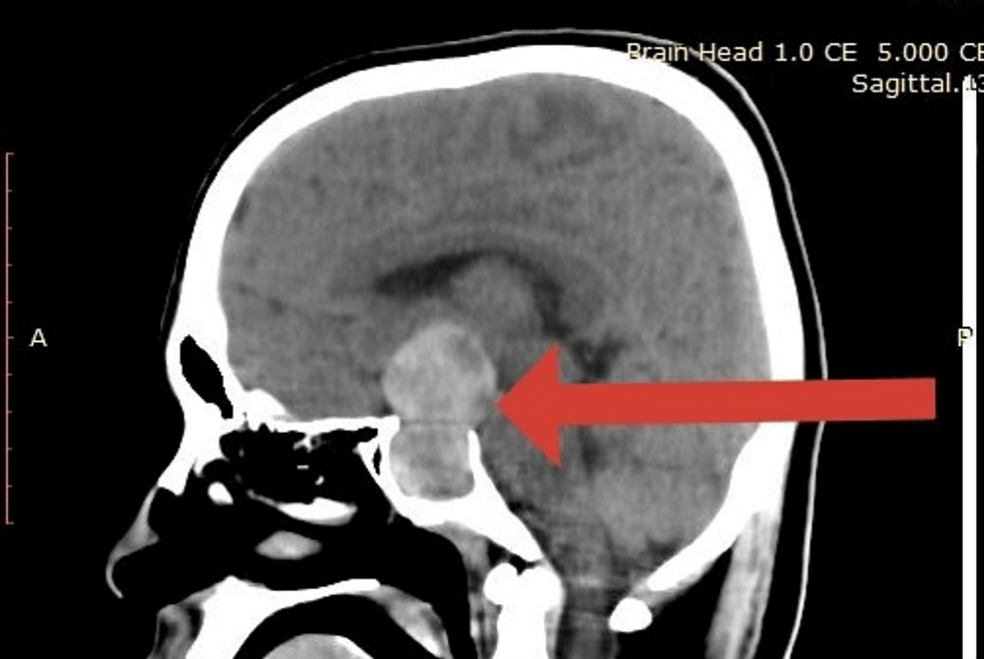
Non-contrast computed tomography (NCCT) brain sagittal view demonstrating a hyperdense large sellar lesion with hypodensity within the hyperdense area seen. Suprasellar and parasellar extensions were also noted. Indicates a possible pituitary macro adenoma with bleeding into the tumor.
